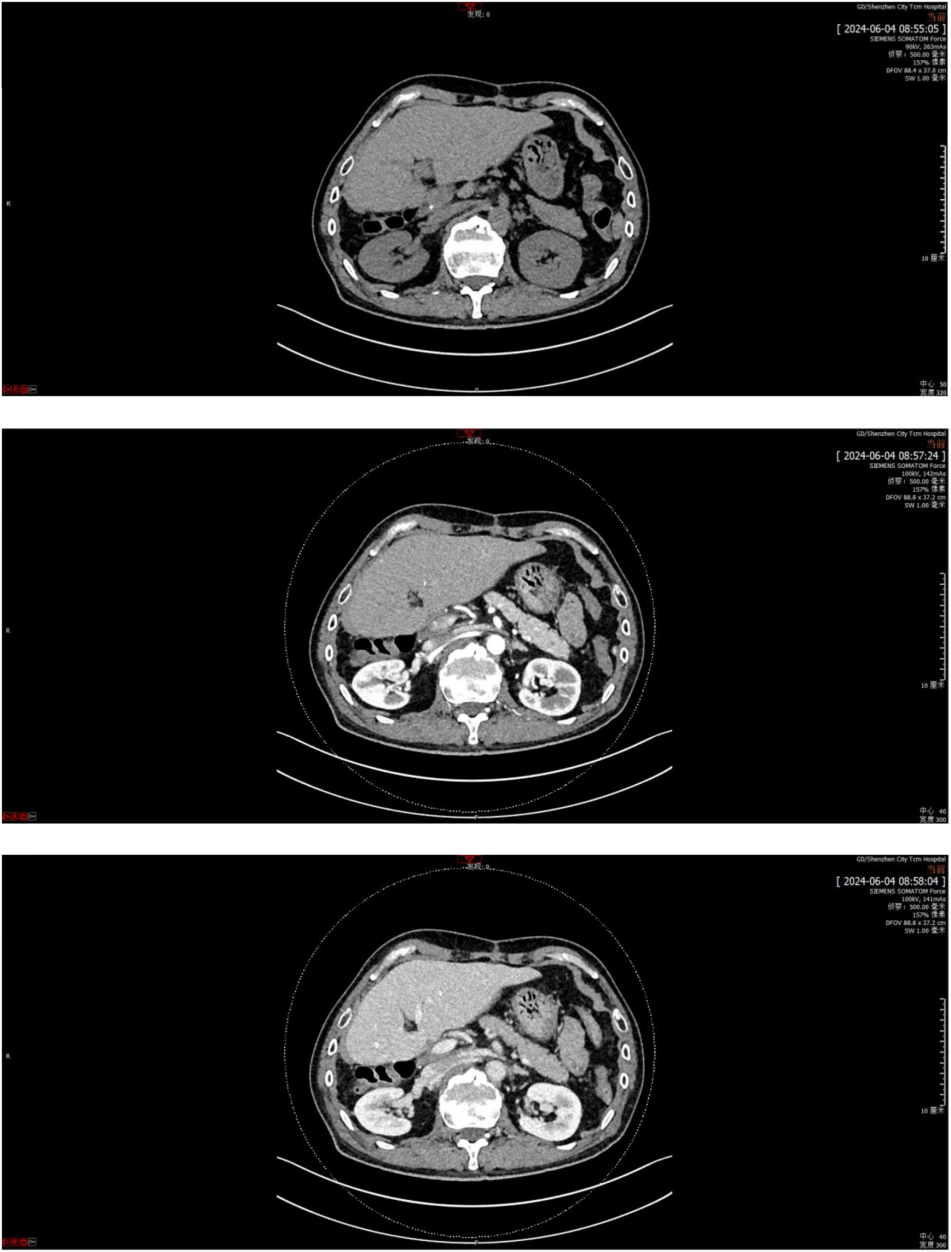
CT scan of the patient at 12 months post-surgery.
